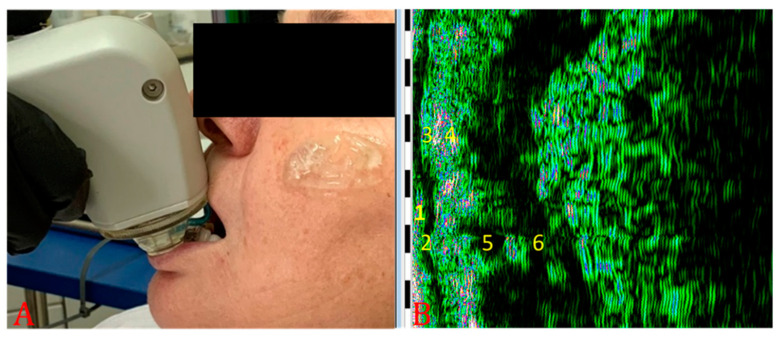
Lower lip HFU examination. (A) Transducer position on inner surface of the lower lip, (B) the lower lip ultrasound structure: 1—superficial layer of the nonkeratinized epithelium, 2—spinosum and basal layers of the nonkeratinized epithelium, 3—papillary and reticular layers of the lamina propriae, 4—submucosa, 5—minor salivary gland, 6—orbicular oris muscle.
